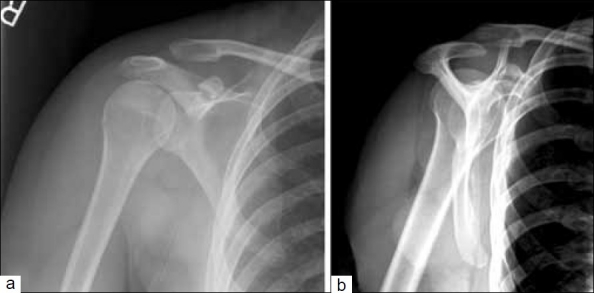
Anteroposterior radiograph (a) of the right shoulder demonstrates a coracoid fracture and type III acromioclavicular (AC) separation. It appears that the fracture is through the base of the coracoid process. The scapular lateral radiograph (b) redemonstrates the injury to the AC joint and visualization of the coracoid fracture
